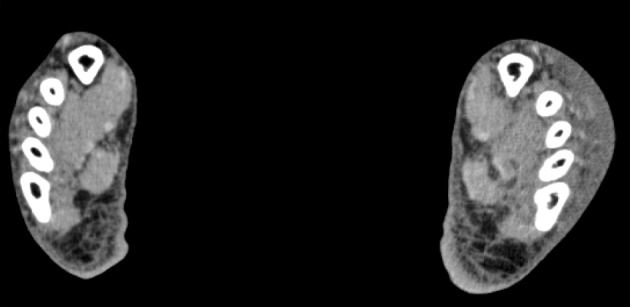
The CT scan of the lower extremities revealed edematous changes in adipose tissue of the upper side of the left foot.
